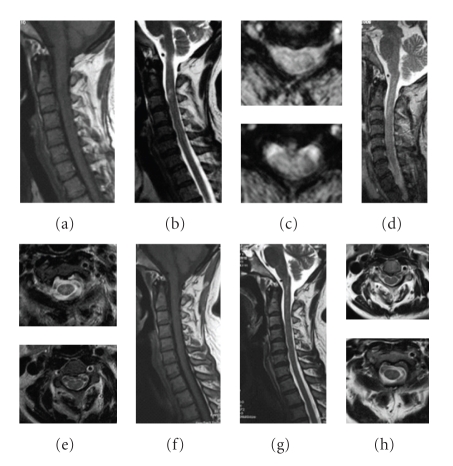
A 51-year-old female suffered from tetraparesis and frequently paroxysmal tics of extremities for 3 months. ATT treatment quickly improved her symptoms. After 2-year ATT, she fully recovered without visible symptoms. The MRI changes are as follows: (a) sagittal T1-weighted MRI showing focal thickening of the cord at C2-C3 level, (b) sagittal T2-weighted MRI showing a focal demyelinating lesion, (c) axial T2-weighted MRI showing the lesion to involve the whole cord, (d) sagittal T2-weighted MRI after 6 months of treatment, showing the swelling of the cervical spinal cord and patchy abnormal signal have resolved, (e) axial T2-weighted MRI showing an abnormal signal remains, (f) sagittal T1-weighted MRI after 2 years of treatment is normal, (g) sagittal T2-weighted MRI after 2 years of treatment revealing no abnormal signals, and (h) axial T2-weighted cord MRI after 2 years of treatment.
